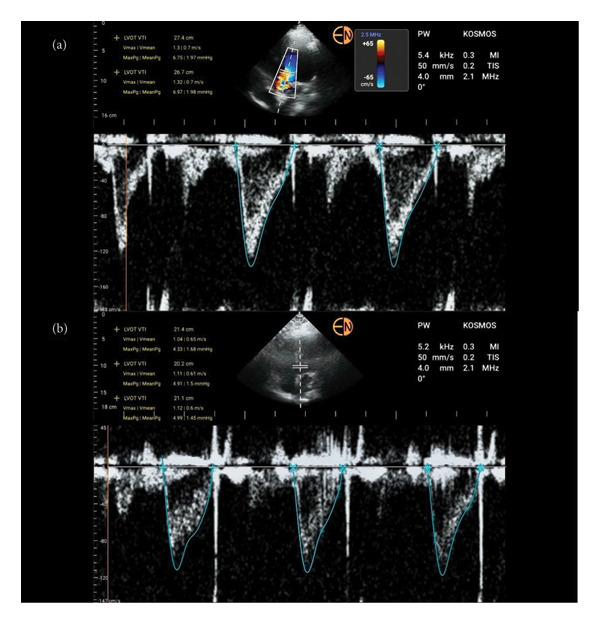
Pulse wave Doppler VTI tracings obtained from the apical five chamber view. (a) VTI obtained intraoperatively which showed an average VTI of 27 cm. (b) VTI obtained 4 hours after end of surgery which showed an average VTI of 20 cm.
